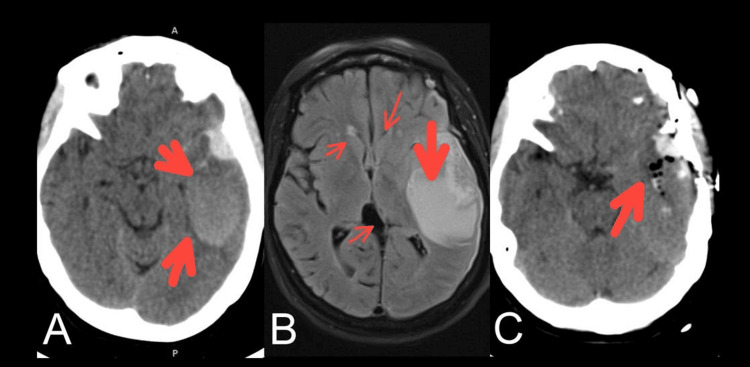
Brain radiological images A: Axial Brain CT scan showing SICH with acute and subacute components, subarachnoid hemorrhage, mass effect on the temporal horn and uncus. B: Brain MRI-Flair sequence showing the thin rim of subdural hematoma and subarachnoid hemorrhage in the Sylvian fissure (midline shift is more appreciated) and edema. C: Postoperative axial Brain CT scan showing SICH evacuation and improvement of mass effect on the uncus. SICH: Spontaneous Intracranial Hemorrhage
